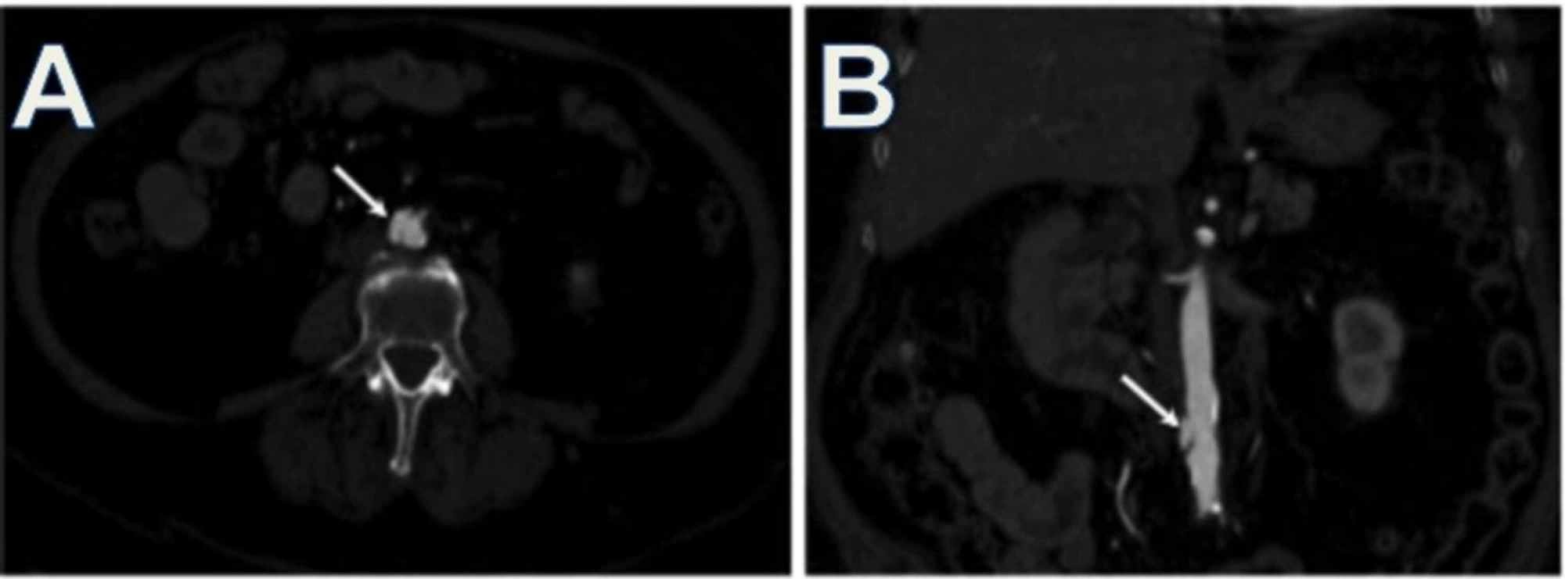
Penetrating Atherosclerotic Ulcer Axial image (A) and coronal reformat (B) of the contrast-enhanced CT demonstrate an atheromatous plaque in the abdominal aorta extending beyond the intima into the aortic media. PAU will increase the patient’s risk of intramural hemorrhage, pseudoaneurysm, or dissection formation.
